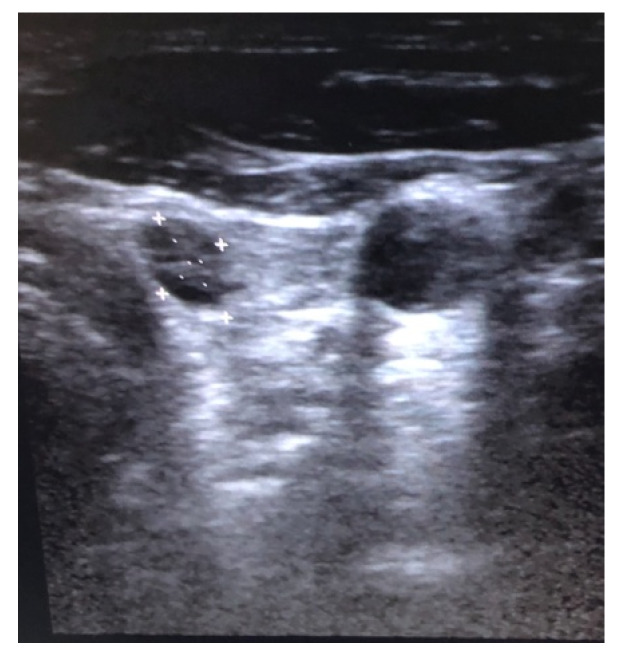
Thyroid ultrasound revealing a left lobe hypoechoic thyroid nodule.
